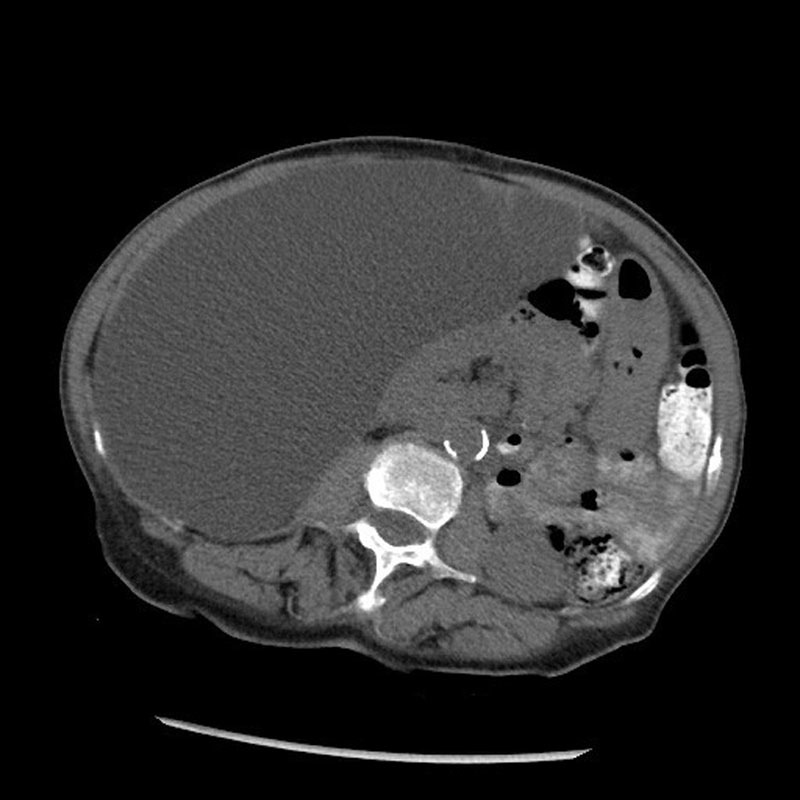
Abdominal computed tomography shows a large right retroperitoneal cystic lesion with displacement of the right kidney and bowel loops to the left.
